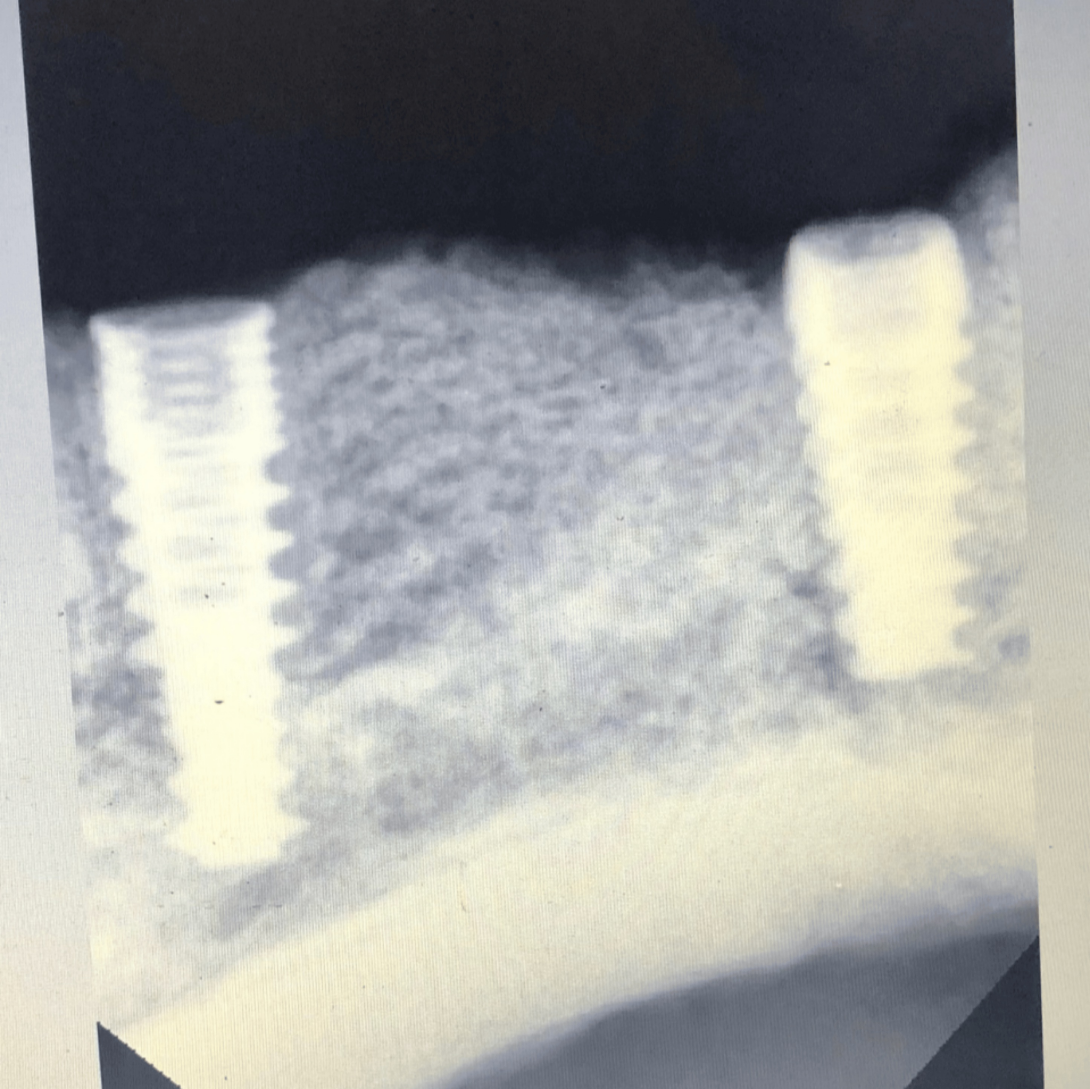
Radiograph immediately after implant placement.
